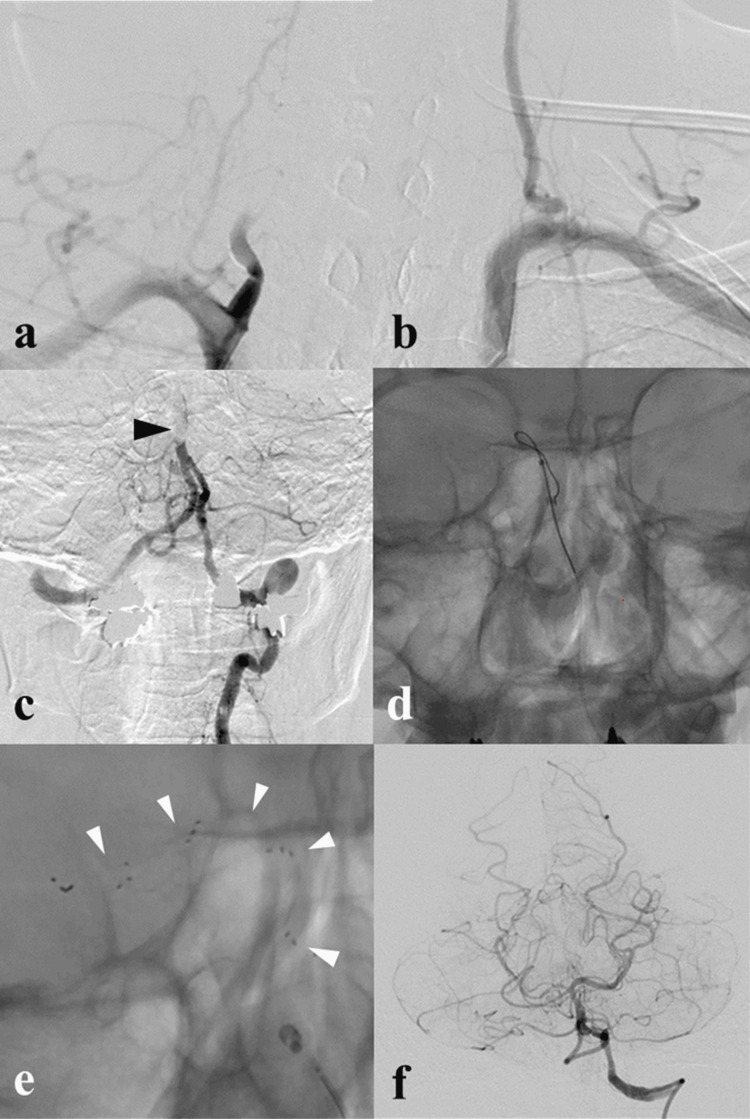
Imaging findings in the mechanical thrombectomy (a) Right brachiocephalic angiogram showing occlusion of the right vertebral artery (VA). (b) Left subclavian angiogram showing an intact left VA. (c) Left vertebral angiogram showing occlusion of the basilar artery (BA, black arrowhead). (d) X-ray image showing a micro guidewire passed through the occlusion. (e) X-ray image showing the stent retriever deployed from the posterior cerebral artery (PCA) to BA (white arrowhead). (f) Left vertebral angiogram showing complete BA recanalization after mechanical thrombectomy (MT).
